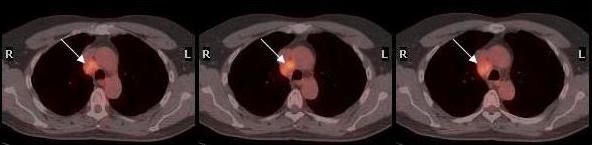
PET scan of thorax. ^18^F-FDG PET scan of the thorax showing a 2.2 cm enlarged right paratracheal lymph node.
